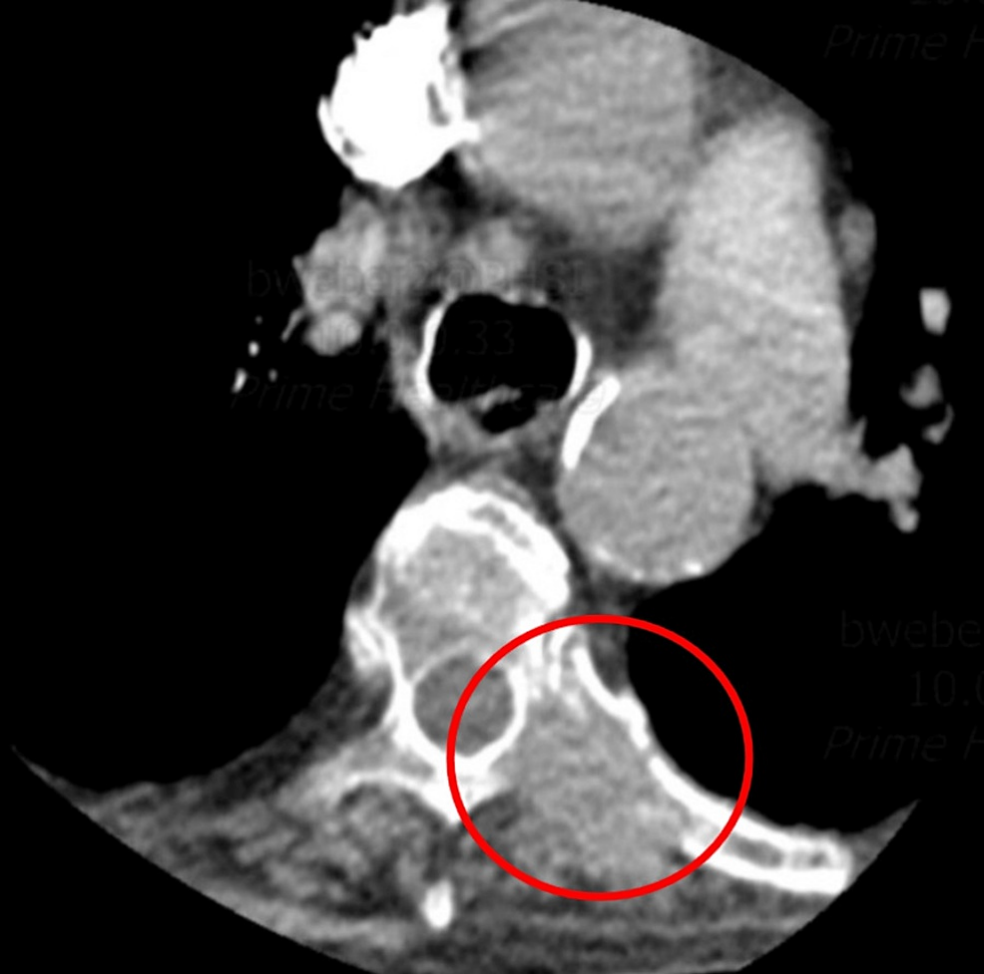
Computed tomography of the thoracic spine depicting possible osseous metastases to the ribs (red circle).
